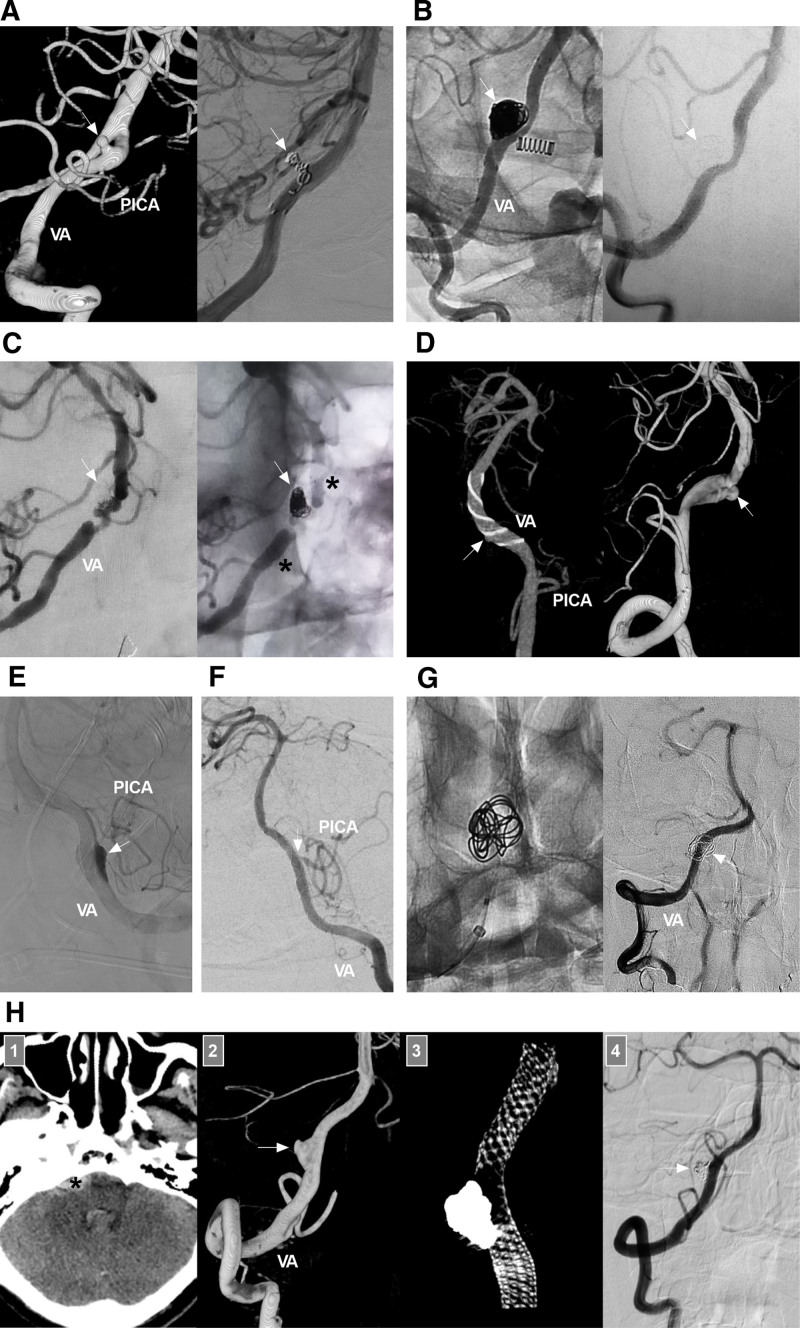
Reconstructive treatment for intracranial VA dissecting aneurysms without PICA involvement. (A) Traditional stent-assisted coiling: left panel: DSA image showing a small dissecting aneurysm of the VA post-PICA (arrow); right panel: DSA image showing coiling of the aneurysm (arrow) with stenting assistance. (B) Traditional stent-assisted coiling: left panel: unsubtracted DSA image showing a large VA dissecting aneurysm (arrow) that has been coiled with stenting assistance; right panel: follow-up DSA image showing cure of the aneurysm (arrow). (C) Neuroform Atlas stent-assisted coiling: left panel: DSA image showing a VA dissecting aneurysm (arrow) that has been coiled with stenting assistance; the parent VA has multiple tandem stenoses; right panel: unsubtracted DSA image showing the coiling (arrow) and stent markers (asterisks). (D) Recurrent VA dissecting aneurysm: left panel: reconstructive DSA image showing a VA dissecting aneurysm (arrow) covered by a LEO stent; right panel: follow-up DSA image showing aneurysm (arrow) recurrence. (E) FD deployment alone: DSA image showing contrast agent retention (arrow) in the aneurysm after FD deployment. (F) DSA image showing a narrowed PICA at the origin (arrow) after FD deployment. (G) FD deployment with loose coiling: left panel: X-ray image showing a VA dissecting aneurysm treated by FD deployment with loose coiling; right panel, follow-up DSA image showing cure of the aneurysm (arrow). (H) Ruptured aneurysm treated with FD deployment and coiling: panel 1: computed tomography image showing subarachnoid hemorrhage (asterisk); Panel 2: DSA image showing a VA aneurysm (arrow); Panel 3: vaso-reconstructive image showing the FD and coil; panel 4: follow-up DSA image showing that the aneurysm (arrow) has been cured. DSA = digital subtraction angiography, FD = flow diverter, PICA = posterior inferior cerebellar artery, VA = vertebral artery.
